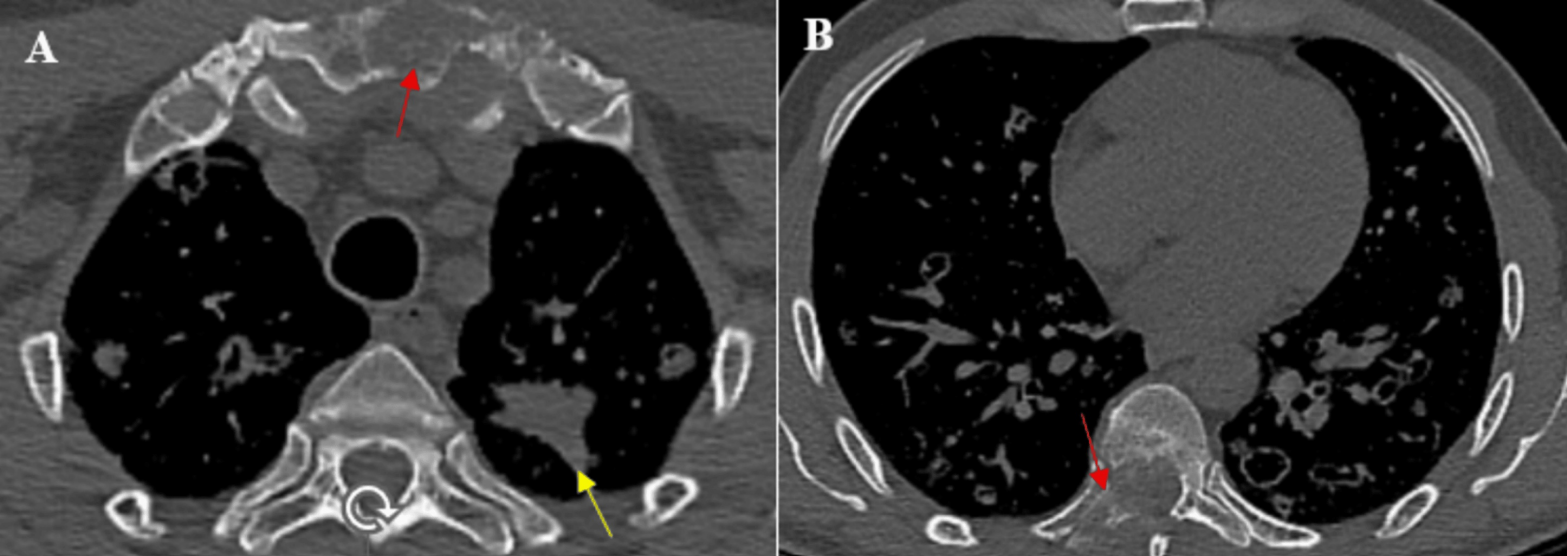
Axial chest CT images (bone window) (A) Osteolytic lesion of the sternal manubrium (red arrow) associated with left upper lobe consolidation (yellow arrow).
